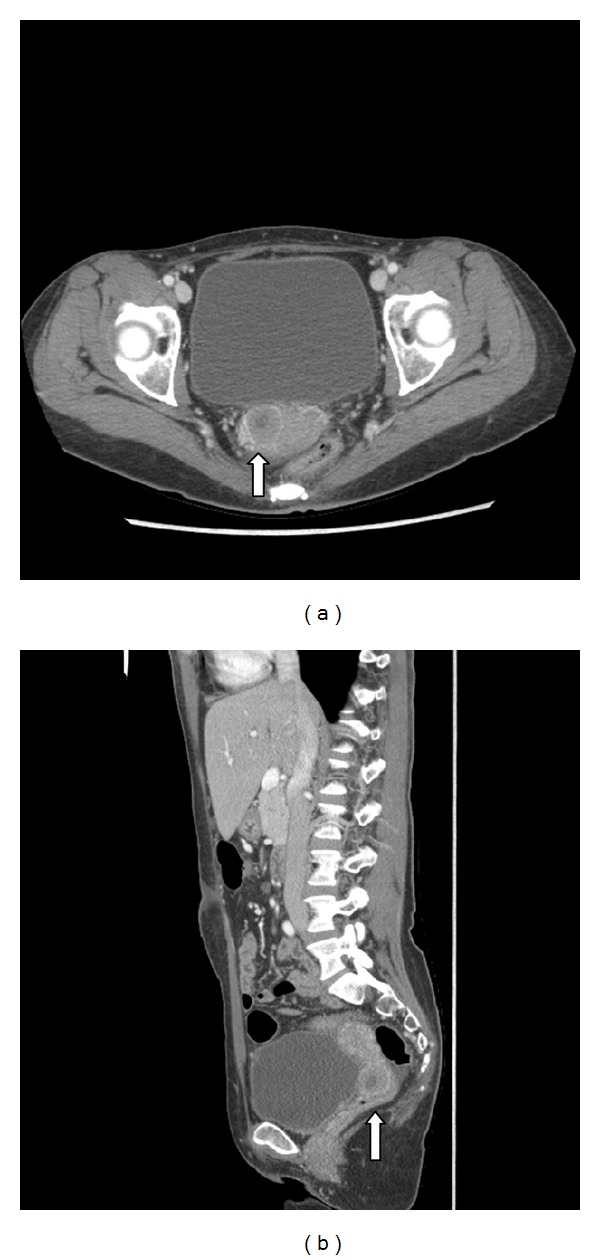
Axial (a) and sagittal (b) postcontrast CT of the pelvis. An enhancing cervical 2.3 cm × 2.2 cm mass (arrow) is on the right side of the cervix.
